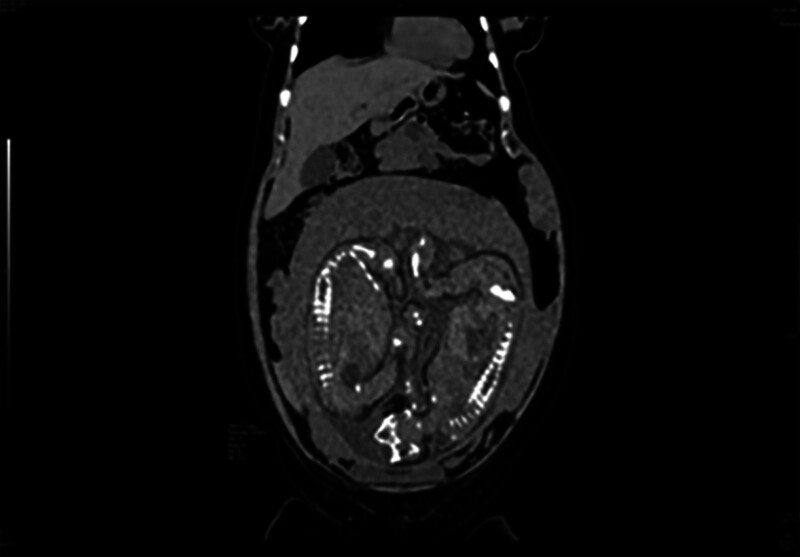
Computed tomography reveals a high probability of pelvic and abdominal hemoperitoneum.
